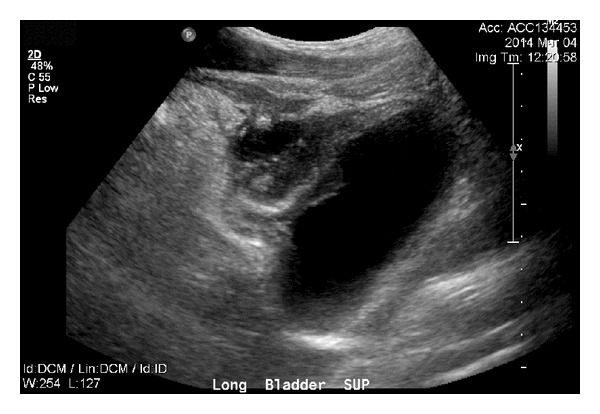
Ultrasound image of 6 cm urachal mass.
